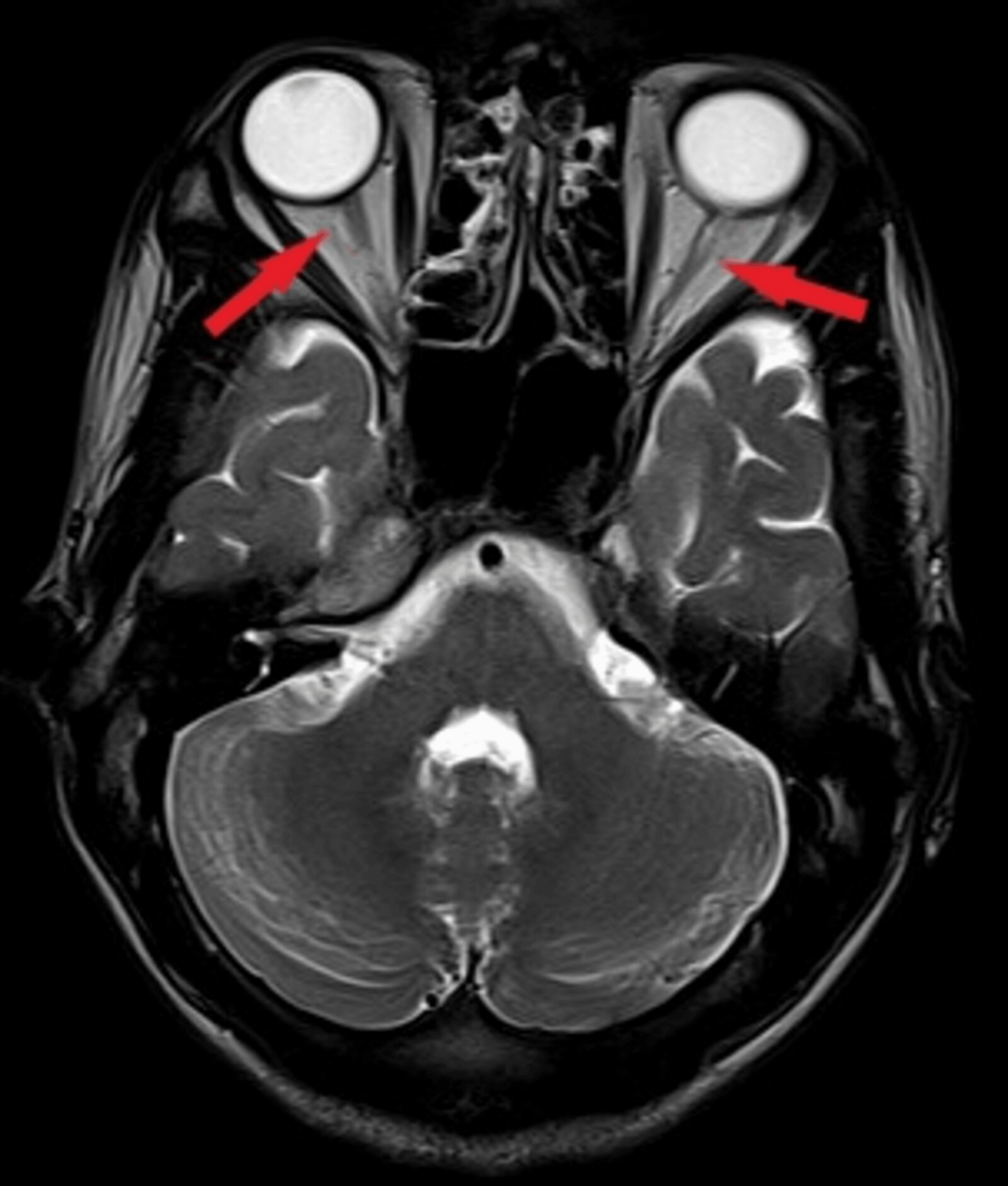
MRI of the brain in T2 axial view showing bilateral optic nerve atrophy (two red arrows). MRI: Magnetic resonance imaging
